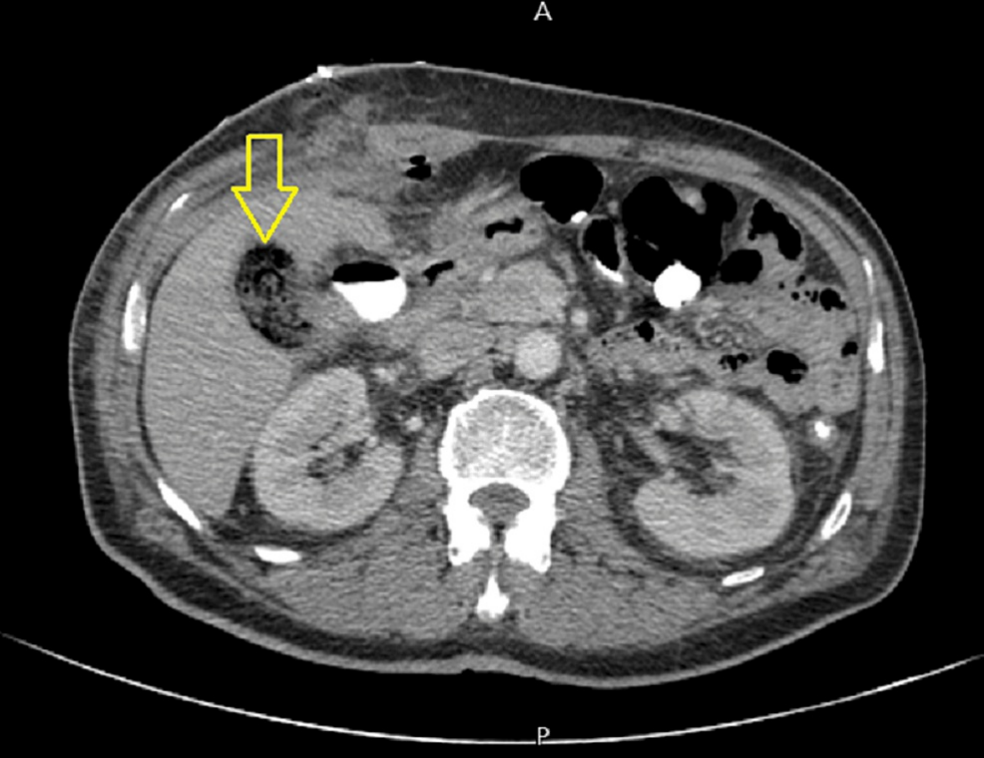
Postoperative abdominal CT with contrast shows mixed gas–fluid attenuation in gallbladder fossa consistent with surgical (normal) findings
